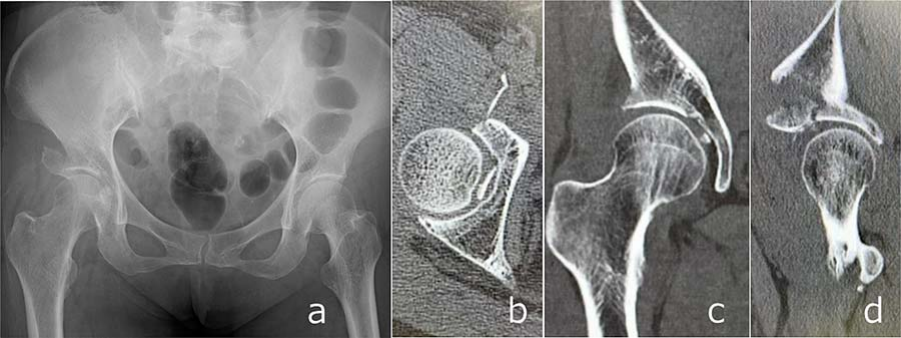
Post-reduction imaging: Anteroposterior radiograph (a) and CT images (b–d) demonstrating reduction of the hip joint with an intra-articular femoral head fracture fragment and anterior acetabular wall involvement.
